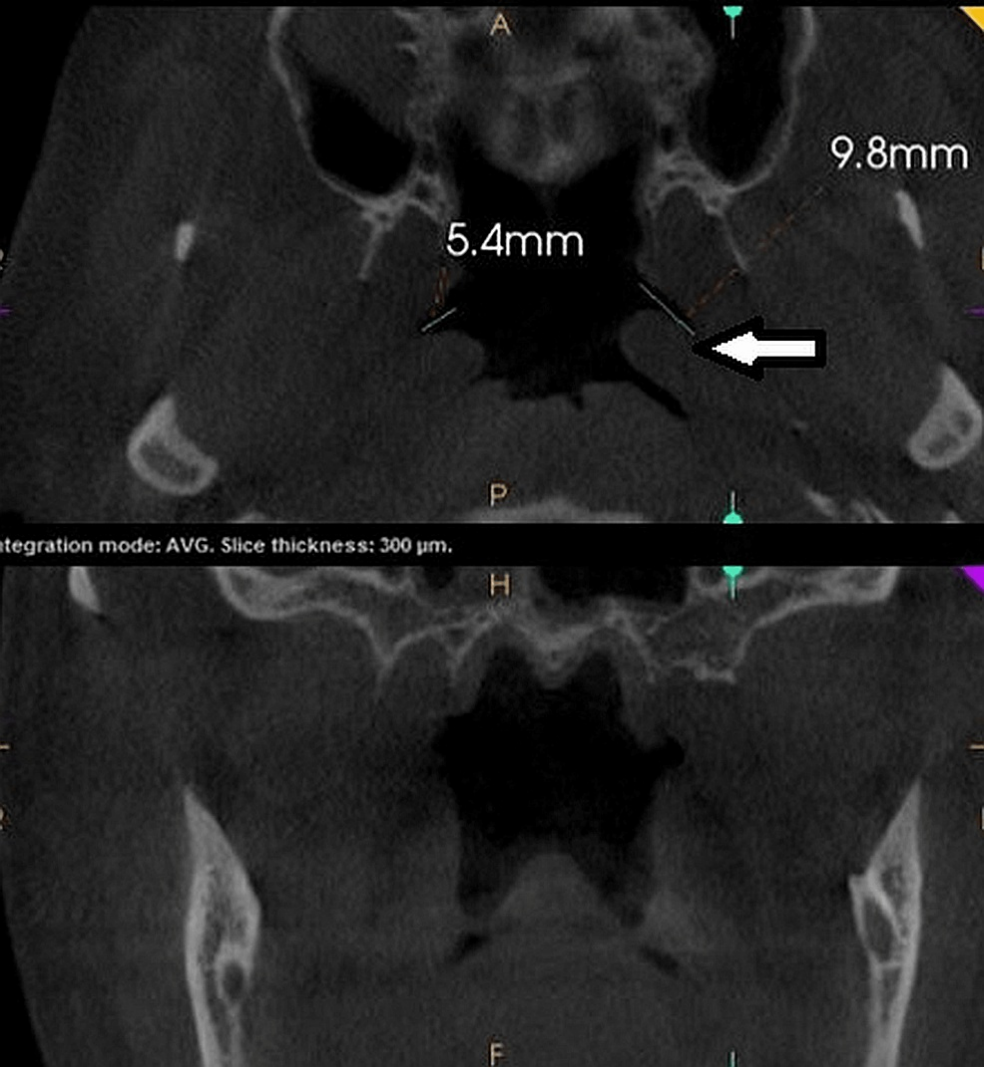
CBCT PNS view to evaluate the eustachian tube
